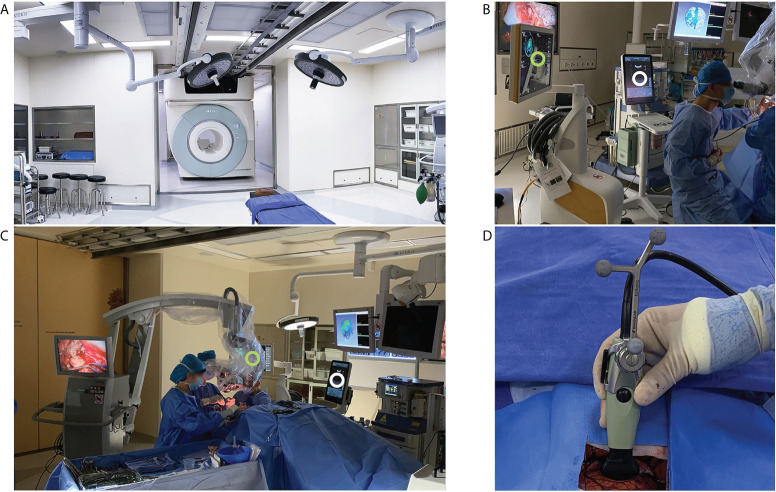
iMRI and iUS systems used in this study. (A) the iMRI system integrated a movable 3.0T MRI scanner. With the shielded door open, the scanner could move into the operating room to perform an iMRI scan. (B) iUS (white circle) and the navigation system (green circle) were registered during surgery to produce 3D iUS and co-planar MRI images. (C) During surgery, the shielded door was closed to isolate the magnetic field. iUS (white circle) and navigation system (Green circle) were placed on the left side of the surgeon. (D) iUS was registered with the navigation system by attaching a rigid reference frame to the transducer.
